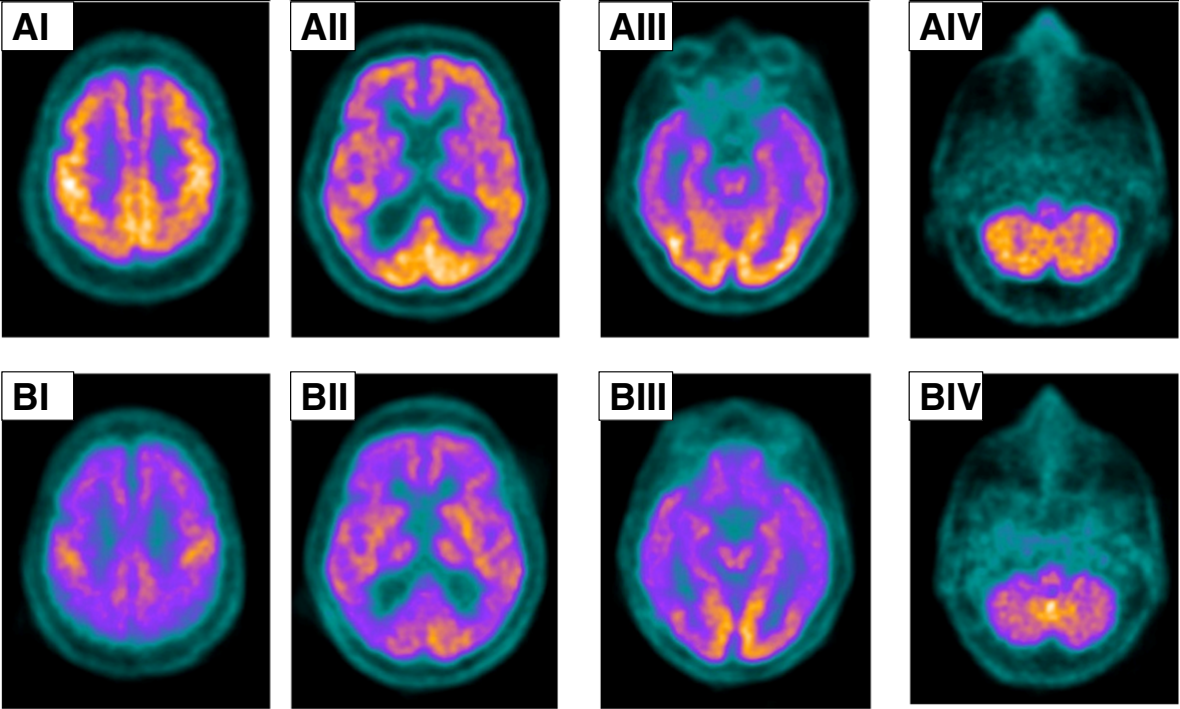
Cerebral scans (axial slices, I–IV) obtained by 2-deoxy-2-[fluorine-18]fluoro-D-glucose integrated with computed tomography-positron emission tomography. Cerebral 2-deoxy-2-[fluorine-18]fluoro-D-glucose integrated with computed tomography-positron emission tomography was performed 23 months before the patient’s death. a Four representative axial slices (AI–AIV) show decreased fluorodeoxyglucose uptake in the frontal and temporal lobes and normal uptake in the posterior cingulum and occipital cortex. Note that the hypometabolism in the affected regions is greater in the right hemisphere. b Cerebral 2-deoxy-2-[fluorine-18]fluoro-D-glucose integrated with computed tomography-positron emission tomography performed 3 months before the patient’s death shows the general reduction in radiotracer uptake in the same axial levels (BI–BIV)
